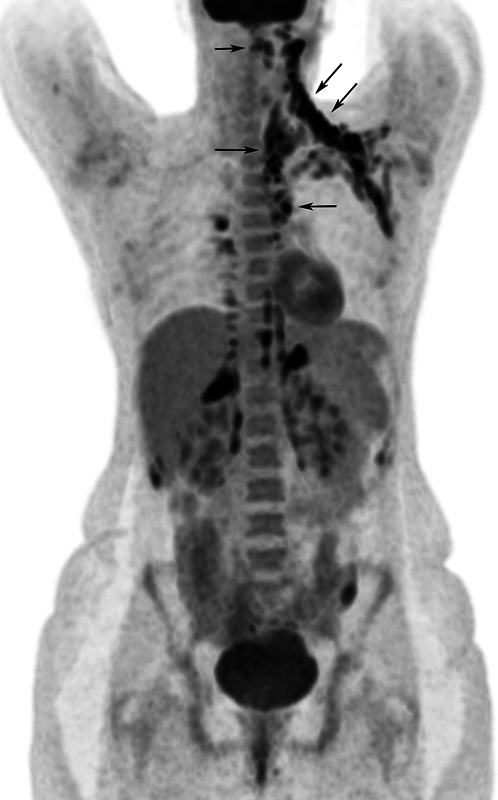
Maximum intensity projection (MIP) showing left-sided focal increased left-sided FDG uptake in cervical, supraclavicular, and thoracic sites (black arrows), mimicking possible malignant disease.
